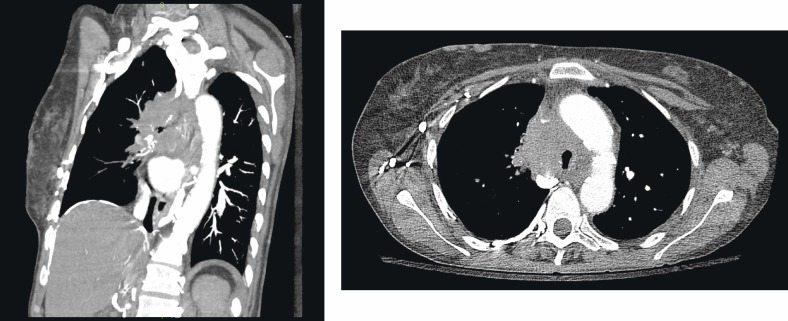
a) CT thorax demonstrating tracheal compression at the level of the tumor b) Lateral view showing the large mediastinal NSCLC with compression of the right mainstem bronchus.
